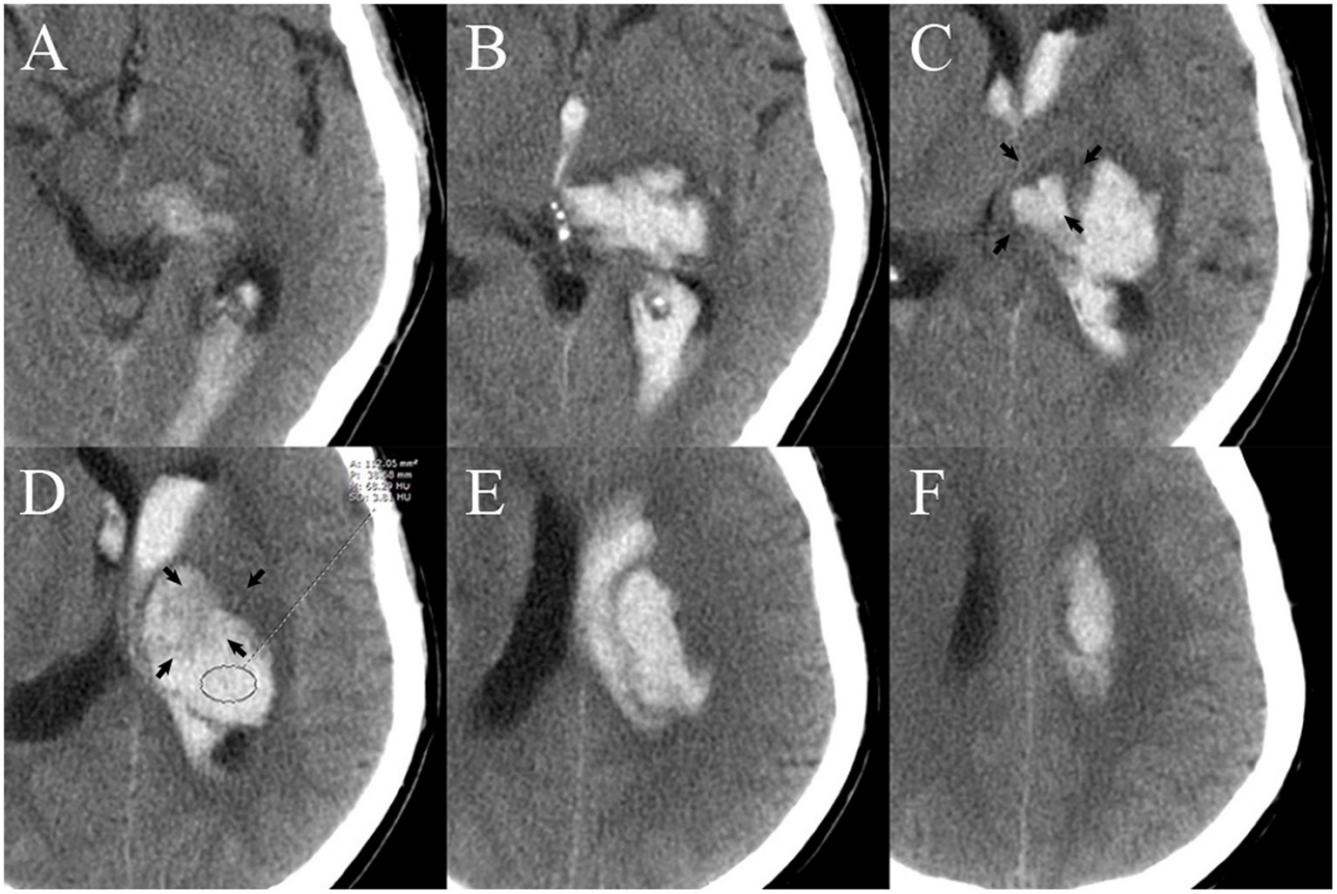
Measurement of the attenuation value of the region of interest (ROI) of the non-hyperdense region of the hematoma. There are six layers of hematoma on axial NCCT images. The upper (A,B) and lower layers (E,F) are not suitable for measurement because they are adjacent to normal brain tissue. The core two layers (C,D) are selected as the best layer for measurement. The area of layer (D) (black arrow) that is adjacent to normal tissue [layer (C), black arrow] may cause partial volume effects that affect the accuracy of the measurement and is therefore excluded from the ROI. A, area; P, perimeter; M, mean; SD, standard deviance; HU, Hounsfield units; NCCT, non-contrast computed tomography.
